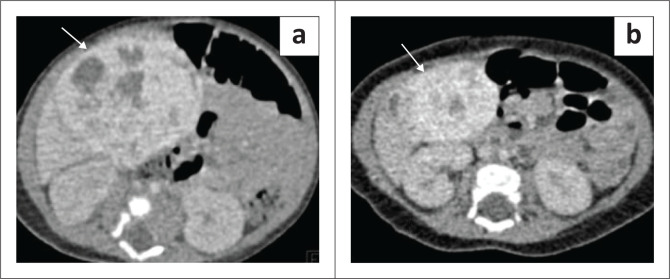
Congenital hepatic haemangioma in a 3-month-old female. Axial contrast-enhanced CT (CECT) through the abdomen shows a large exophytic heterogeneous lesion in the right lobe of liver (arrow in a). Follow-up CECT after 3 months showed significant spontaneous decrease in the size of the mass (b).
